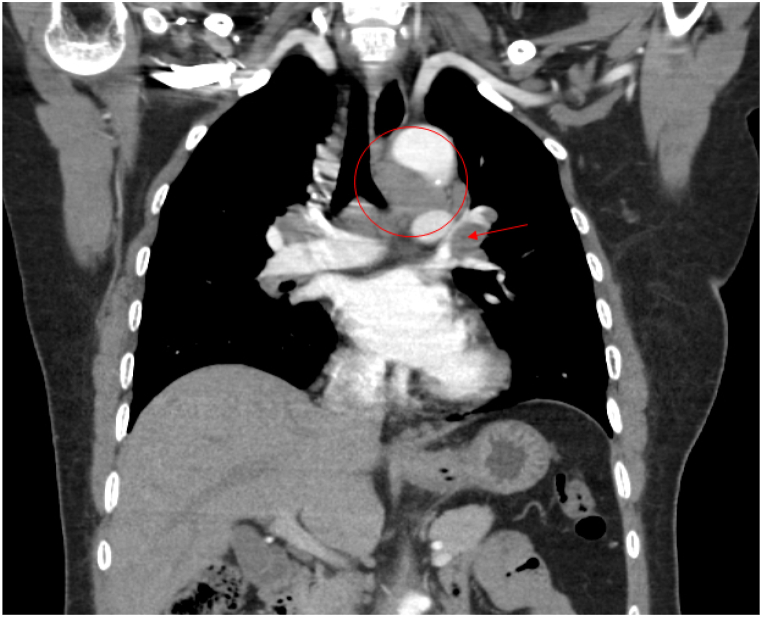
Longitudinal section of computed tomography scan of the chest showing mediastinal lymphadenopathy abutting the esophagus.
